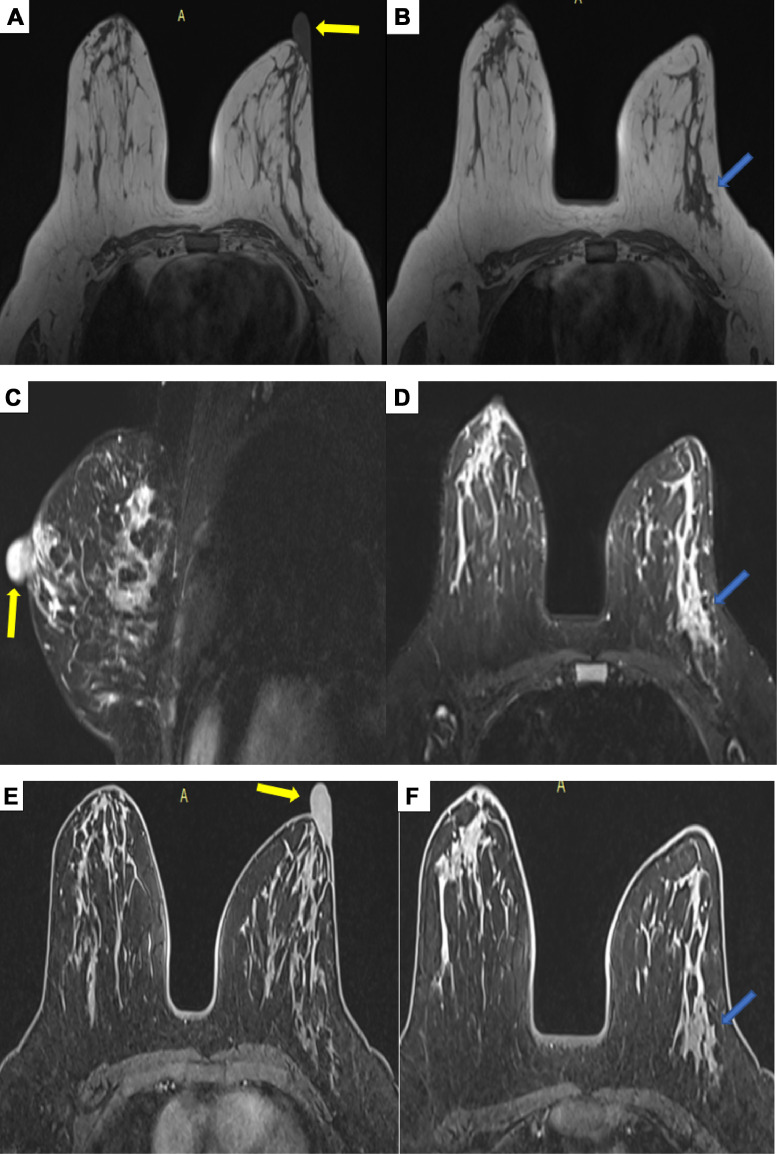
Breast MRI. Axial T1 sequences without contrast injection (A and B), T2 Dixon sequences with fat saturation, sagittal cut (C) and axial cut (D), T1 postcontrast sequences with fat saturation, axial cuts (E and F). Finely heterogeneous thickening of the left nipple, enhanced following contrast injection, measuring 24 × 16 mm (yellow arrow). Furthermore, a tubular lesion was observed in the inferolateral quadrant of the left breast. This lesion, aligned in a ductal orientation, appeared isointense on T1-weighted sequences and hyperintense on T2-weighted sequences, with enhancement following a type 3 curve (rapid uptake followed by washout), characteristic of suspicious lesions. The lesion measured 5.5 × 5.5 × 13 mm (blue arrow).
